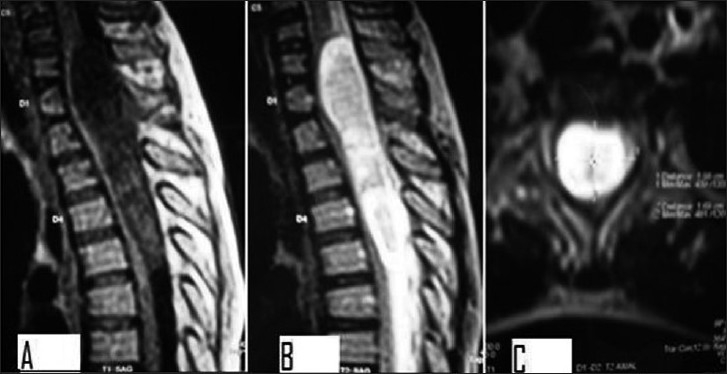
T1-weighted and T2-weighted Magnetic resonance image of the epidermoid cyst with cervico-dorsal extension, the lesion being hypointense on T1 sequence (A) and hyperintense on T2 sequence (B, C)
